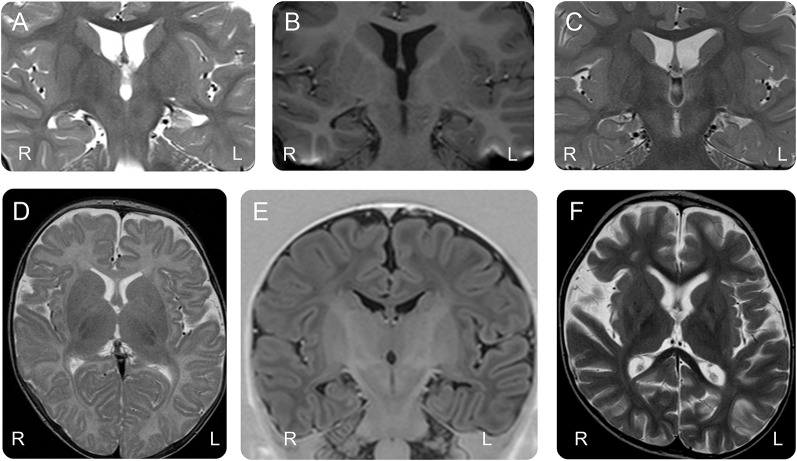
MRI in children with early infantile *SCN1A* encephalopathy (A–C) Coronal views in case 1 (3 years of age), case 9 (5 years of age), and case 2 (1 year of age) show bilateral small hippocampi. (A) Case 1 developed left hippocampal sclerosis. (C) Case 2 has a malrotated left hippocampus. (D, F) Axial T2 and coronal T1 images of case 9 show (D) normal white matter volume at 2 months of age with (F) moderately severe volume loss at 2 years. (E) Coronal views of the hippocampi at 2 months show bilateral small hippocampi with left hippocampal malrotation.
